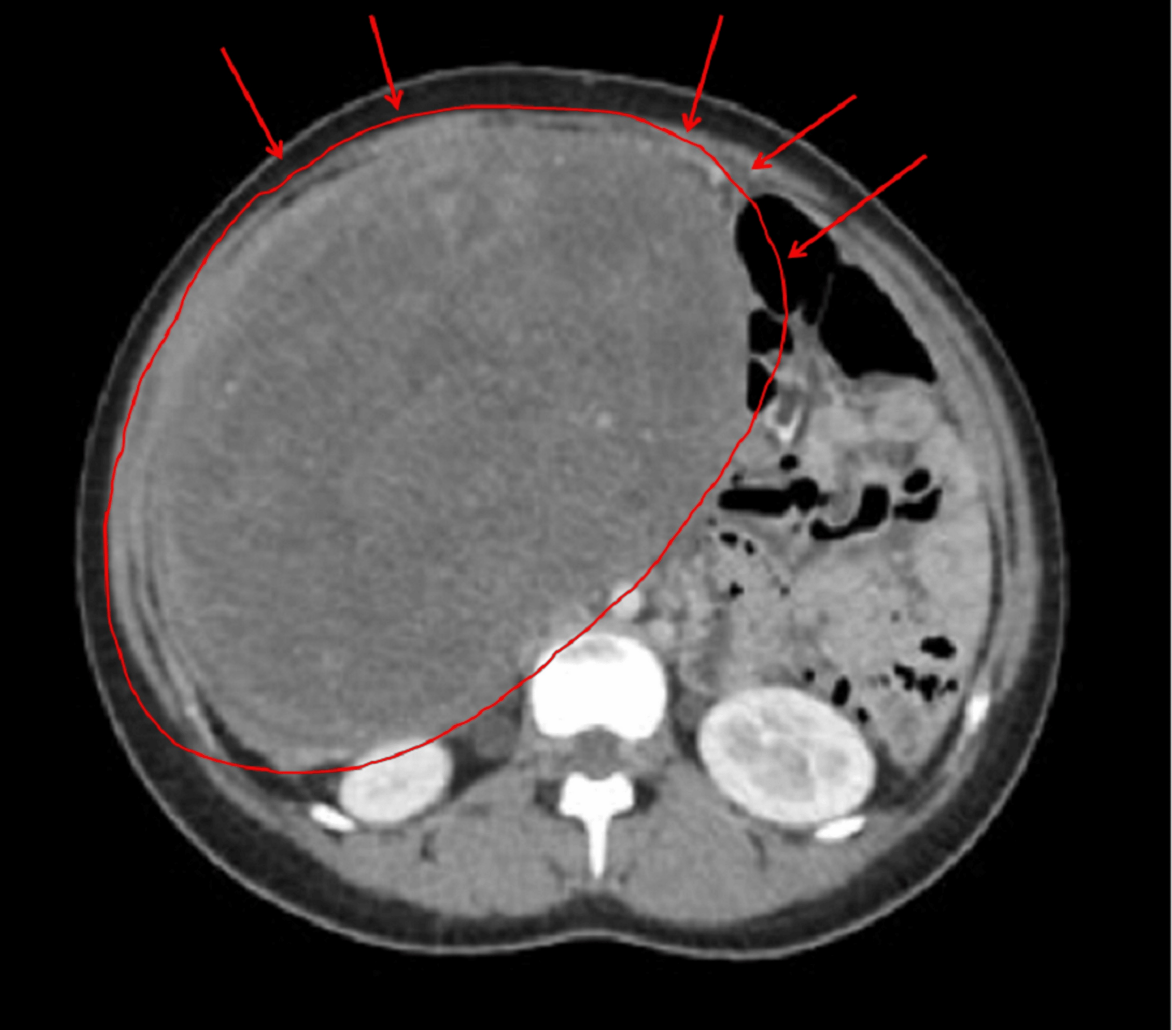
Axial CT scan showing the central location and enhancement of the mass (Case 2)
